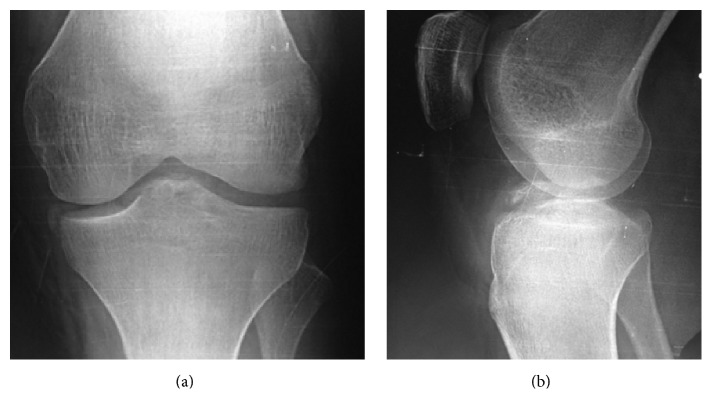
Anteroposterior (a) and lateral (b) preoperative radiographs showing tibial eminence and reverse Segond fractures.
